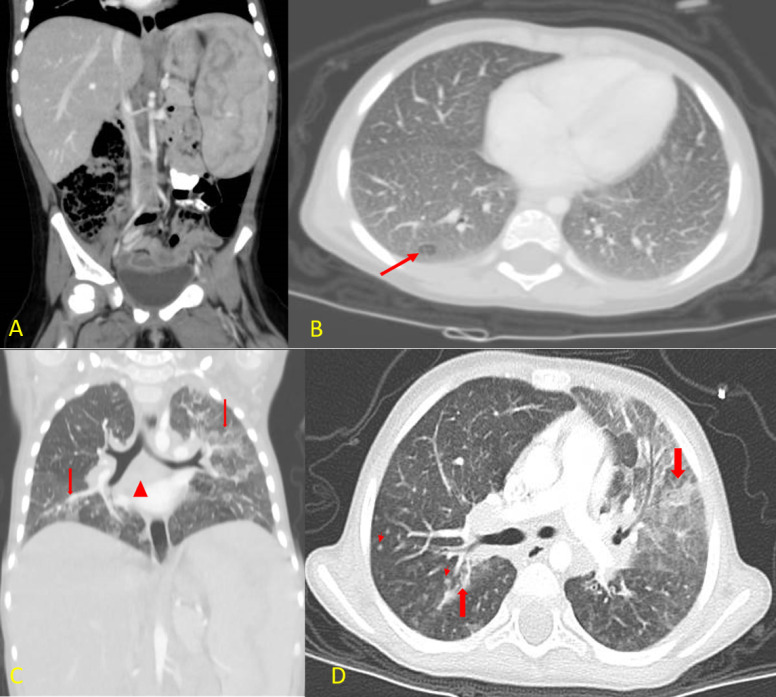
Patient’s CT scans in both coronal (A,C) and axial (B,D) views. (A) Contrasted CT scan of the abdomen and pelvis showing hepatosplenomegaly. (B) Axial lung window settings for lung bases demonstrating diffuse ground-glass opacity of both lung fields with diffuse smooth interstitial thickening and mosaic pattern of lung attenuation (arrow). (C) Coronal CT reconstruction demonstrating again the areas of consolidation, ground-glass opacity, and smooth interstitial thickening in both lung fields (thick arrows). The mediastinal lymphadenopathy, especially in the subcarinal region (arrowhead). (D) Axial CT scan of the chest with IV contrast lung window settings demonstrating multiple areas of consolidations (thick arrows) with surrounding ground-glass opacity, which were more prominent in the left upper lung lobe. There is also diffuse smooth interstitial thickening, and multiple small intraparenchymal lung nodules can be seen (short arrows).
